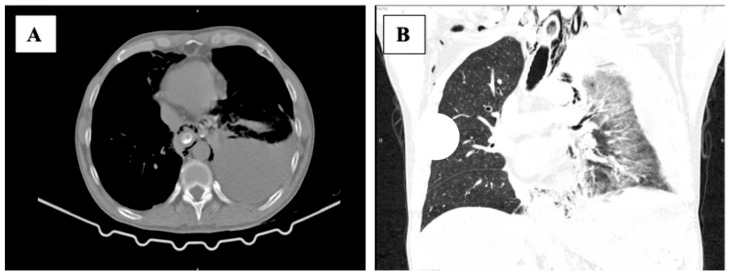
(A) Transverse CT scan of the chest, revealing moderate pneumomediastinum, especially in the posterior mediastinum. (B) Longitudinal CT section indicates left pleural effusion.
